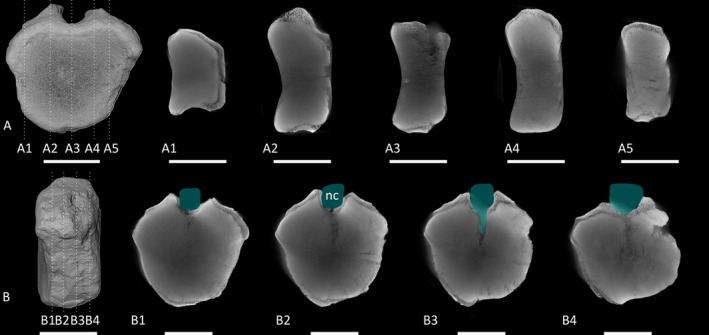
CT scan of *Cetiosaurus* sp. anterior caudal vertebra OUMNH PAL‐J.50308. (A, B) 3D rendering (A) anterior view, (B) left lateral view. (A1–A5) CT scan slices in parasagittal cross‐section. (B1–B4) CT scan slices in transverse cross‐section. nc, neural canal. Key: blue highlight. Scale bars = 100 mm.
